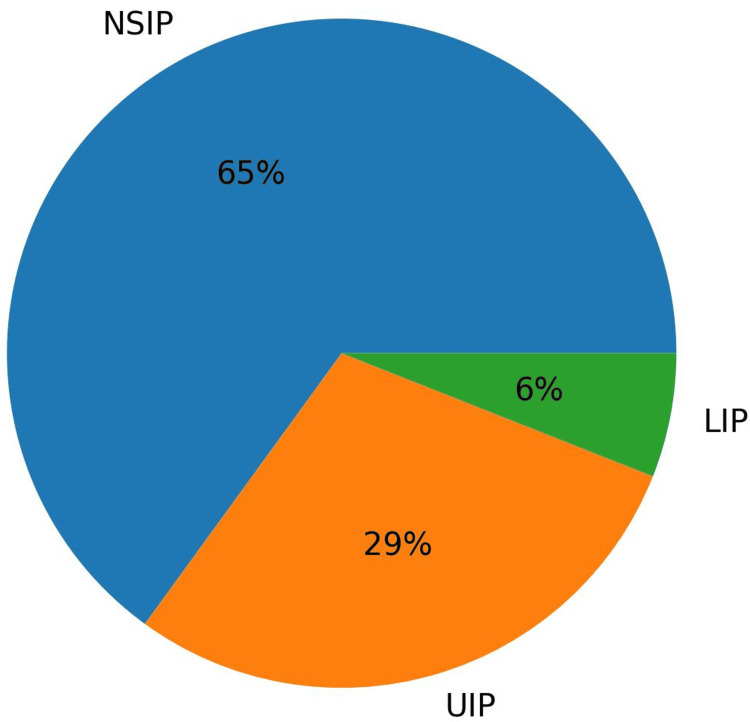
Distribution of HRCT patterns in CTD-ILD The pie chart shows the distribution of HRCT patterns among the study population (total n = 17); NSIP was the most common pattern (65%), followed by UIP (29%) and LIP (6%). HRCT:  High-resolution computed tomography, CTD-ILD: Connective tissue disease–associated interstitial lung disease, NSIP: Nonspecific interstitial pneumonia, UIP: Usual interstitial pneumonia, LIP: Lymphocytic interstitial pneumonia Source: Institutional data from LN Medical College and JK Hospital (Bhopal, MP, IND)
